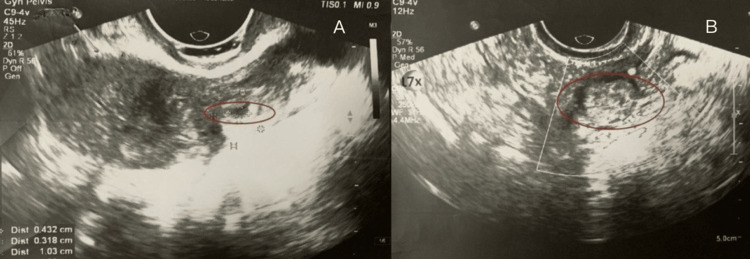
(A) Transvaginal ultrasound: Heterogeneous lesion on day 5 after the second dose of methotrexate. (B) Persistent blood flow noted within the lesion on color Doppler suggesting incomplete resolution
